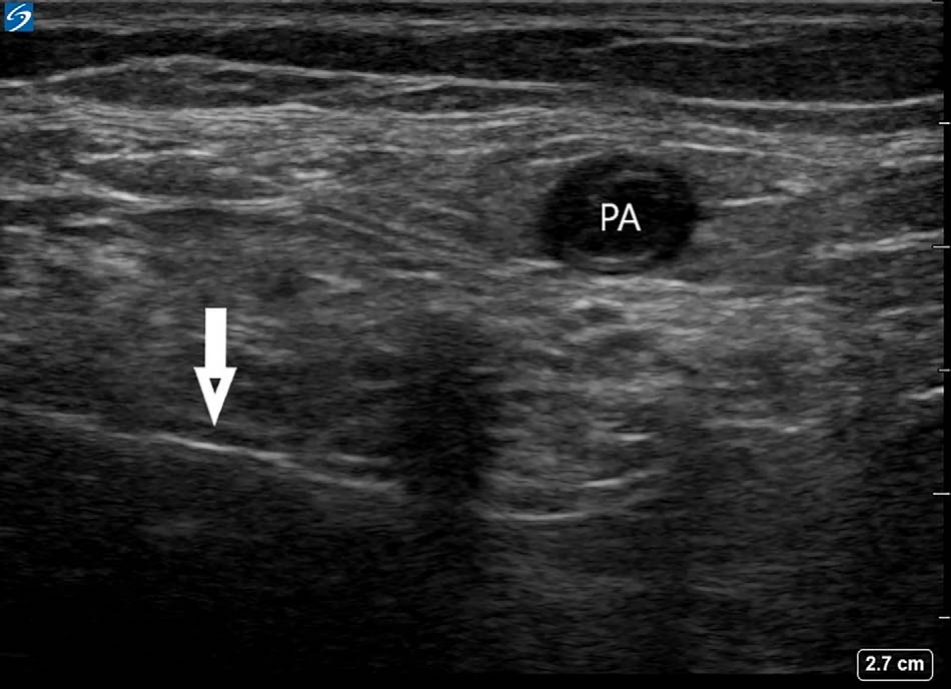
In this image of an ultrasound-guided local anesthetic infiltration between the popliteal artery (PA) and capsule of the knee (iPACK) block, the needle (arrow) can be seen entering from the lateral side into the space between the PA and the femur (out of frame to the right).
